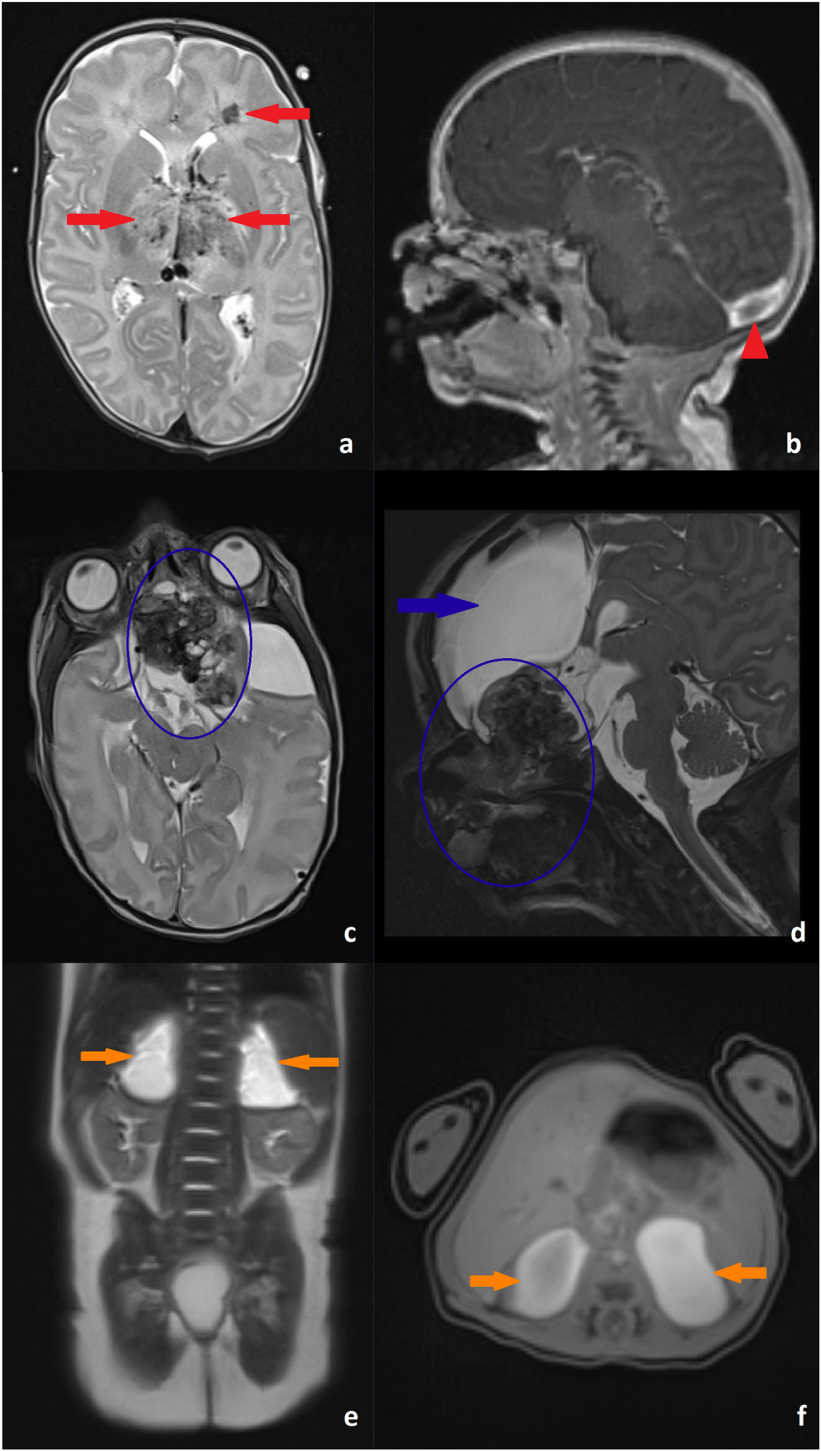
Clinical examples of examinations performed using the FWT. a T2-weighted turbo spin echo sequence demonstrating venous congestion bleeding in the periventricular white matter and basal ganglia (red arrows) due to (b) thrombosis in the sinus confluens (red arrowhead, T1-MPRAGE (Magnetization Prepared Rapid Acquisition with Gradient Echoes) postcontrast) in a 13-day old female patient. T2-weighted turbo spin echo (c) and 3D-SPACE (Sampling Perfection with Application optimized Contrast using different flip angle Evolution) (d) sequence demonstrating a large craniofacial teratoma (blue circles) with a large adjacent intracranial arachnoid cyst (blue arrow) in a 4-day-old female patient. T2-weighted Half fourier Single-shot Turbo spin-Echo (e) and T1-weighted 3D stack of stars gradient echo sequence (f) confirming suspected bilateral adrenal gland bleeding (orange arrows) in a 7-day old male patient
